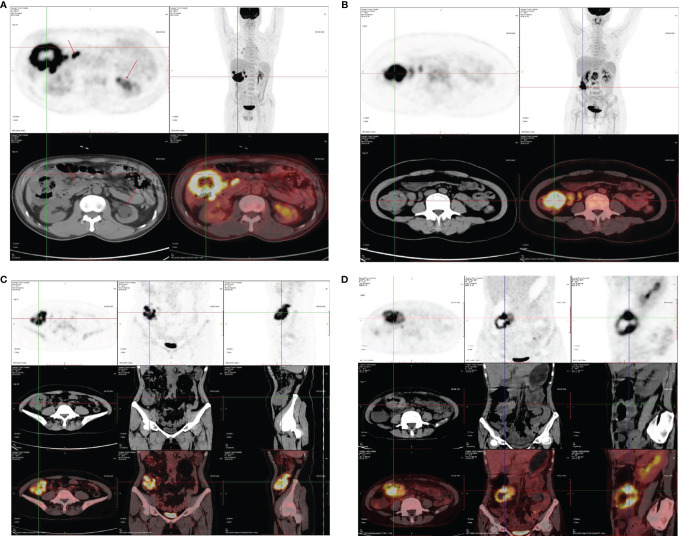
Representative images ^18^FDG-PET/CT scan on CRC patients. (A) Ascending colon, stage IV, SUVmax: 34.0. (B) Ascending colon, stage III, SUVmax: 19.9. (C) Transverse colon, stage II, SUVmax: 21.3. (D) Ascending colon, stage II, SUVmax: 26.1.
